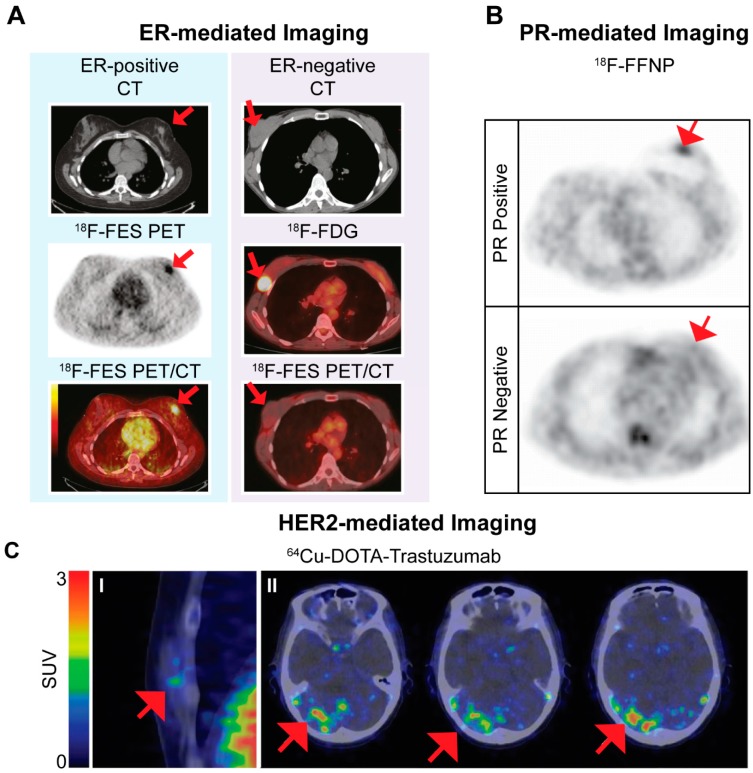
Examples of images using the estrogen receptor (ER)-targeted radiotracer ^18^F-FES (A); progesterone receptor (PR)-targeted radiotracer ^18^F-FFNP (B) and the human epidermal growth factor receptor 2 (HER2)-targeted radiotracer ^64^Cu-DOTA-trastuzumab obtained in breast cancer (BC) patients. Images are adapted from Gemignani et al. [17], Dehdashti et al. [27] and Tamura et al. [31], respectively. (A) ^18^F-FES PET images of an ER-positive and an ER-negative BC lesion. The ER-positive BC lesion visualized by ^18^F-FES PET corresponds with a 2–3 cm lesion seen on CT and is confirmed on the ^18^F-FES PET/CT images. The ER-negative BC lesion is visualized on CT and by ^18^F-FDG PET but shows no ^18^F-FES uptake. This research was originally published in *JNM.*
